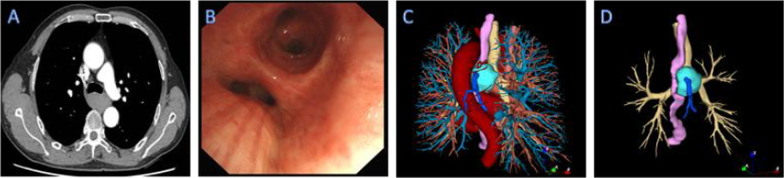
A Chest contrast-enhanced CT revealed that the mass located at the tracheal bifurcation. B Bronchoscopy revealed external pressure stenosis at the bronchial origin of the left lower lobe. C Three-dimensional reconstruction showed that the mass is a hemangioma originating from the azygos vein arch. There were two arteries from the aorta running among the tumor body, the esophagus and the trachea. D The tumor body obviously extruded the esophagus and trachea
